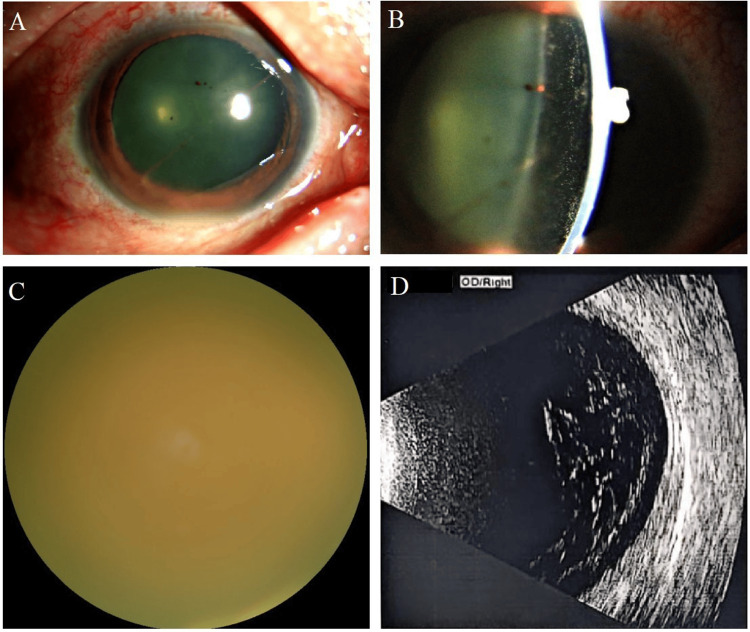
Baseline ophthalmic examination findings of the right eye. (A) Marked ciliary congestion. (B) Slit-lamp examination revealed abundant inflammatory cells and a significant aqueous flare in the anterior chamber. (C) Dense vitreous opacity precluding view of the fundus. (D) Ocular B-scan ultrasound showed extensive vitreous opacities.
